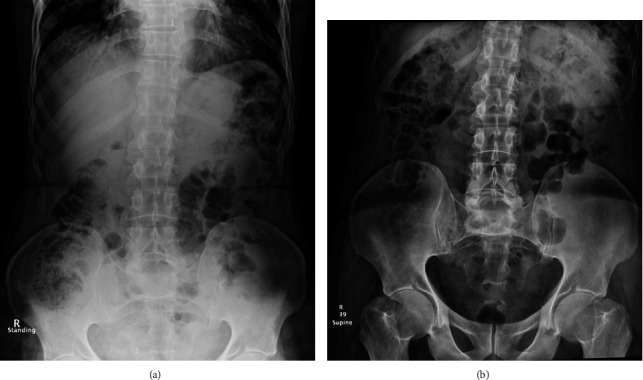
Erect (a) and supine (b) abdominal X-ray radiographs showing colonic fecal and gas content.
